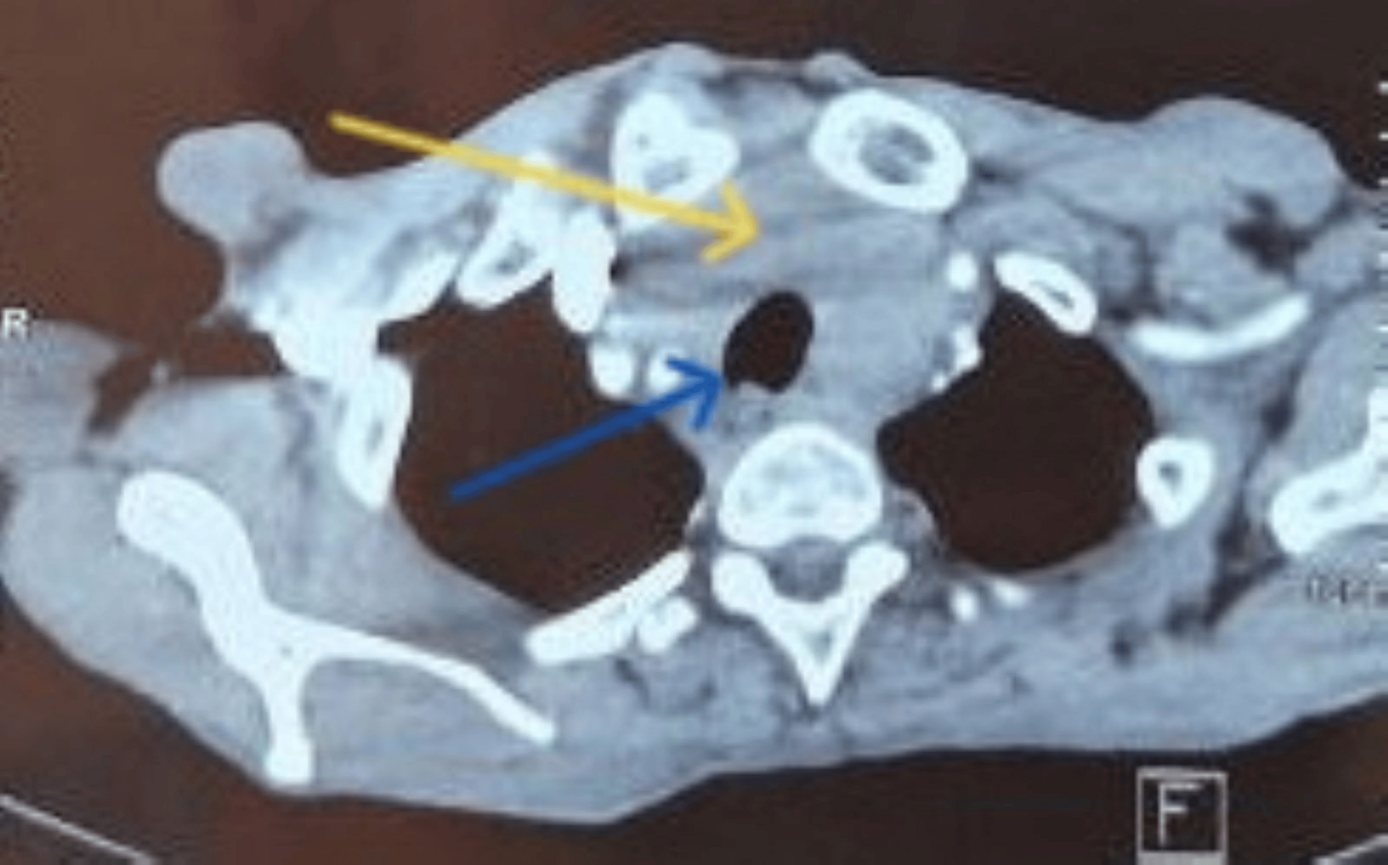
Cervicothoracic CT (axial view) showing a large substernal goiter (yellow arrow) causing a very mild deviation of the trachea (blue arrow)
